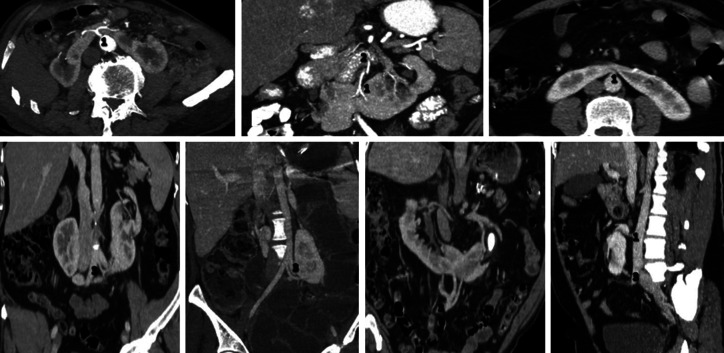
Type 2 accessory artery. (A) Median artery supplying the isthmus of the horseshoe kidney on axial CT image. (B) Median artery supplying the isthmus of the horseshoe kidney on coronal CT image. (C) Median artery supplying the isthmus of the horseshoe kidney on axial CT image. (D, E) Accessory artery originating from the sacral artery feeding the isthmus of the horseshoe kidney on coronal CT image. (F) Accessory artery originating from the sacral artery divides into several branches distally and feeds the isthmus of the horseshoe kidney and the inferior parts of both kidneys on coronal CT image. (G) Accessory arteries originating from both the median and sacral arteries on sagittal CT image.1*- *Accessory artery originating from the median artery; 2-IMA; 3- accessory artery originating from the sacral artery. IMA, inferior mesenteric artery; CT, computed tomography.
